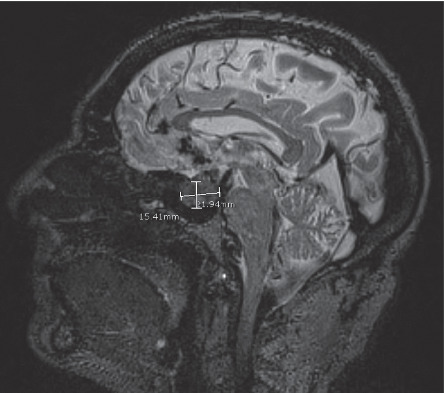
Preoperative sagittal T2-weighted MRI showing 15.41 mm by 12.94 mm paraganglioma in the sellar and suprasellar region.
